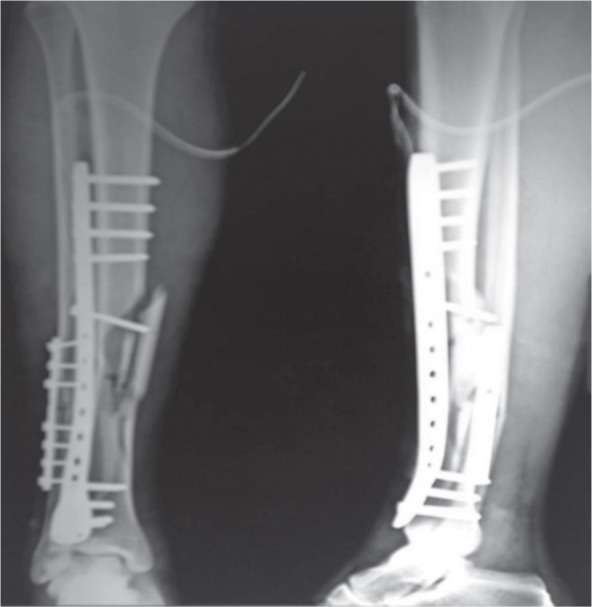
Anteroposterior and Lateral radiograph showing fracture fixation of tibia and fibula of same patient shown in Figure 2.
